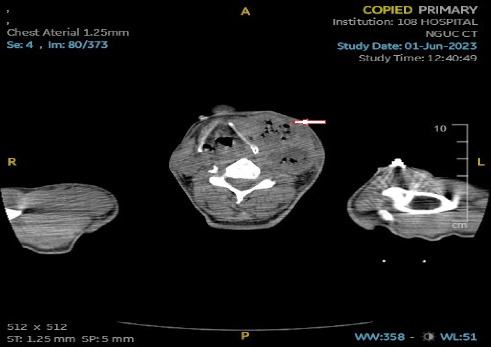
Computed tomography of the left and anterior neck region shows a mass with gas-fluid levels, border is not clear, size 40 × 56 × 106 mm.
